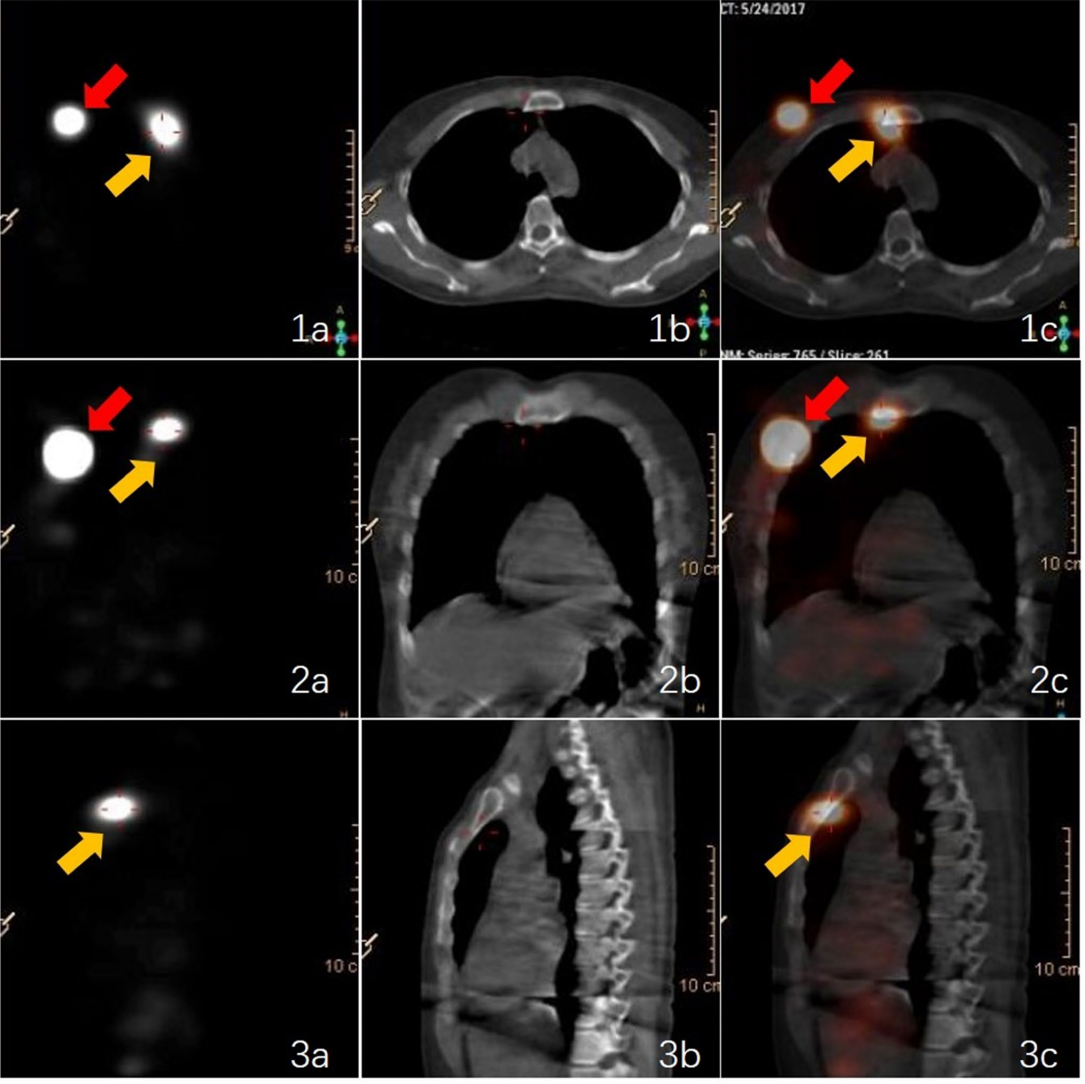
A positive internal mammary lymph node (yellow arrow) detected using single-photon emission computed tomography (a), computed tomography (b), and SPECT/CT (c) viewed in the axial plane (1), coronal plane (2), and sagittal plane (3). The red arrow indicates the injection point
